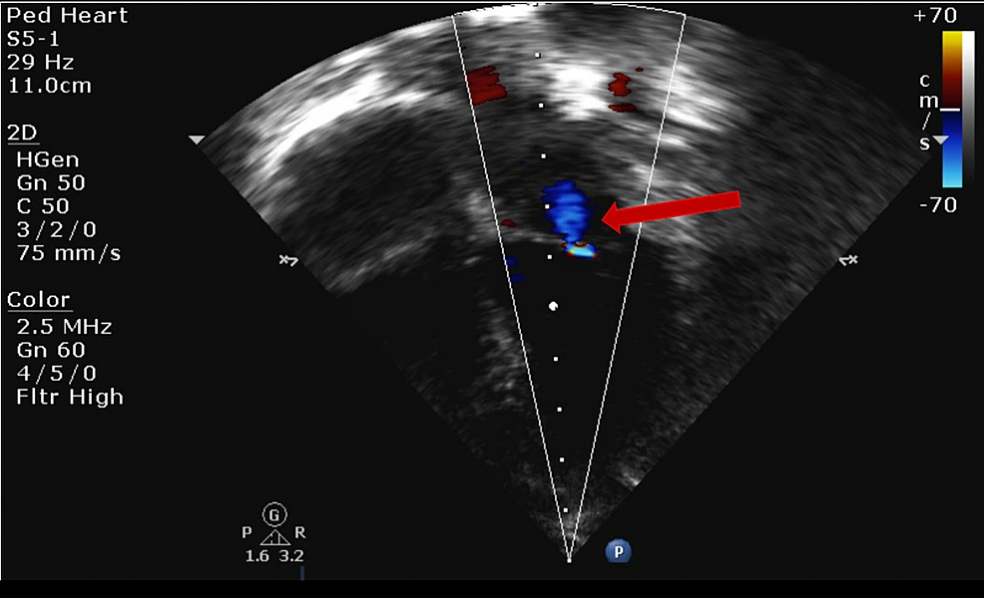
Transthoracic echocardiogram (TTE) apical four-chamber view showing mild mitral regurgitation
